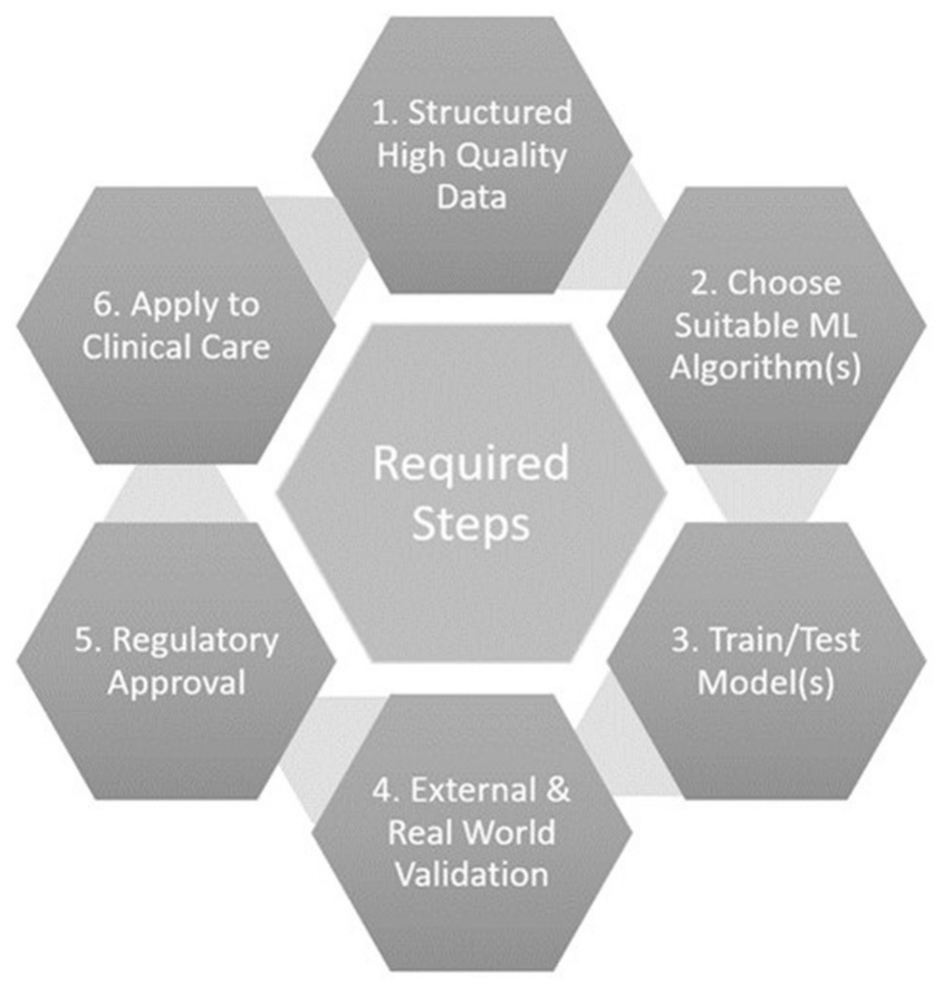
Key steps required for using machine-learning for echocardiographic diastolic analysis.
